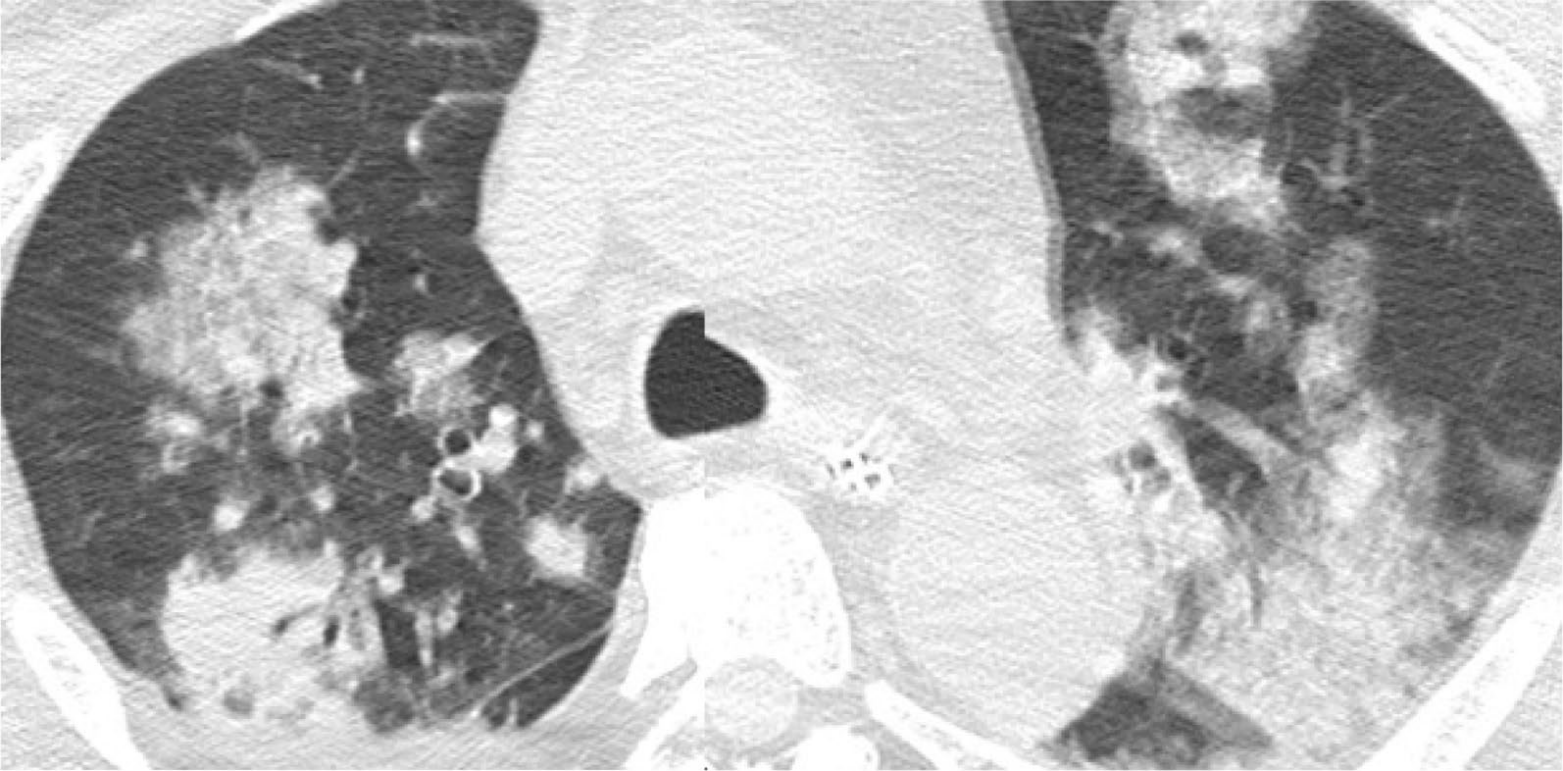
Computed tomography scan performed on postoperative day 1. Nodular shadows with the halo sign were observed
